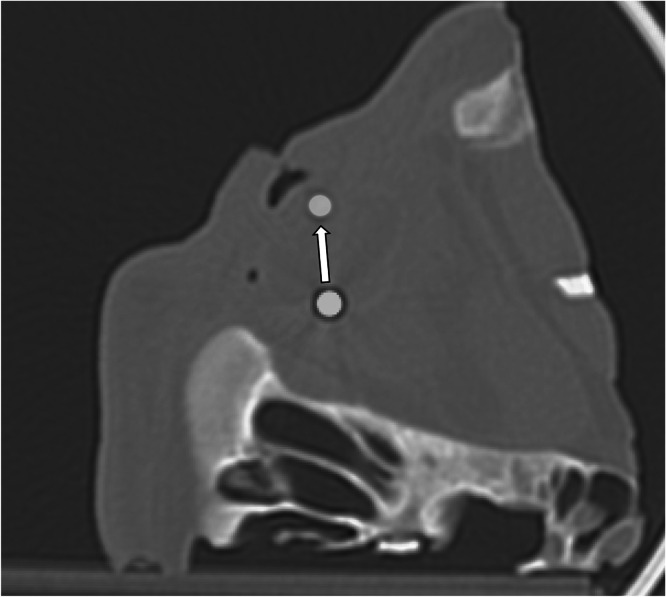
Fusion of pre- and post-MRI CT scans after rigid spatial registration–coronal view. The white arrow shows the displacement of an intravitreal 4-mm ferromagnetic foreign body after MRI scan.
